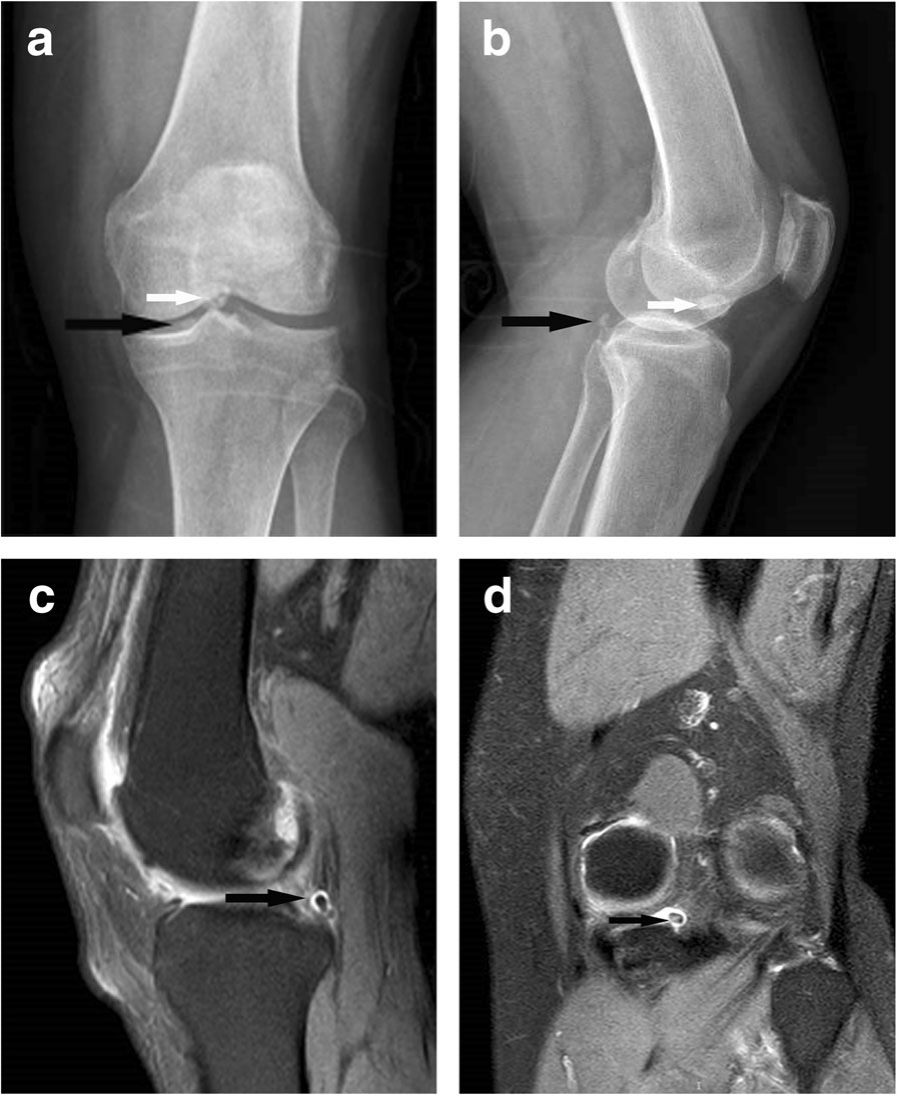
Images of loose bodies in the posteromedial compartment of the knee. One (black arrows) at the medial joint space, the other (white arrows) in the femoral intercondylar notch. a, b Preoperative anteroposterior and lateral views of the radiograph, respectively. c, d Sagittal and coronal views of MRI, respectively
